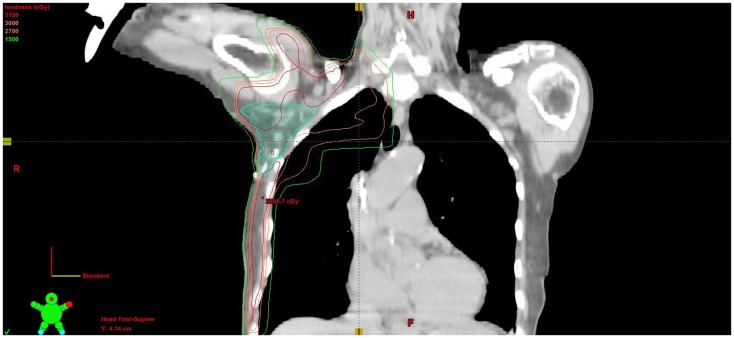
Coronal CT slice of treatment plan showing isodose lines and contoured axillary nodal volume.
